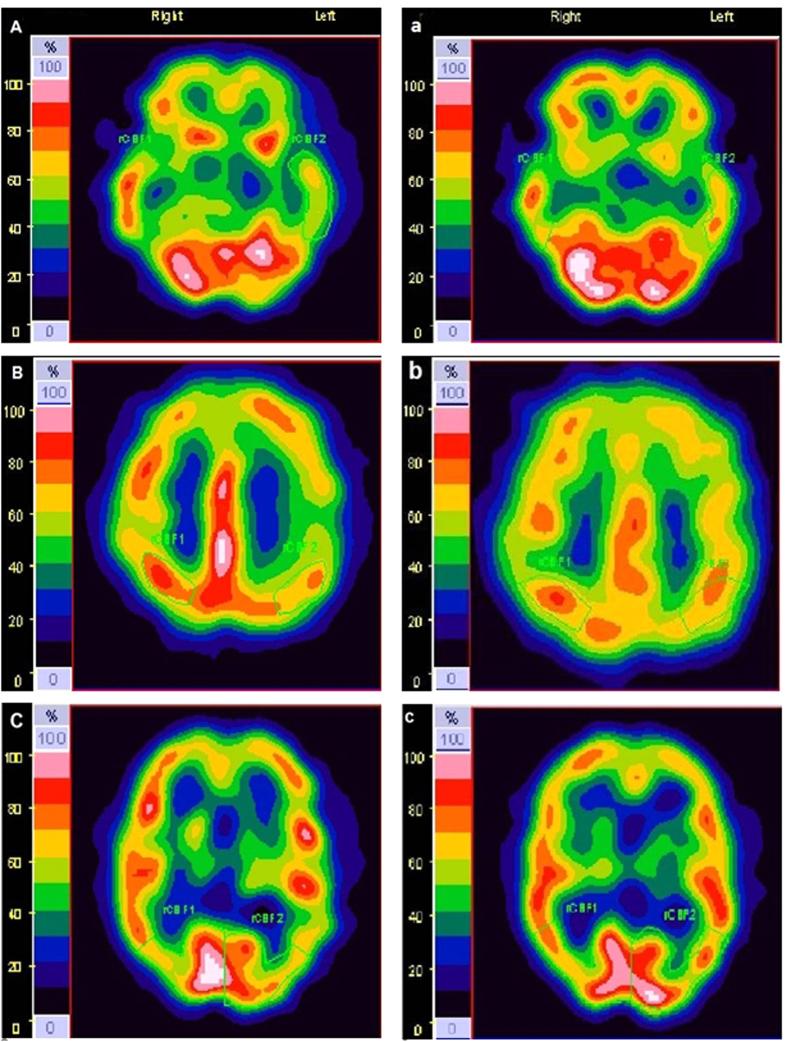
Representative SPECT images before rTMS ((A): temporal lobe; (B) parietal lobe; and (C): occipital lobe), and after rTMS (a–c), showing the areas that displayed a significant increase in rCBF in the patients with ISSHL after the application of low-frequency rTMS treatment over the TAC ipsilateral to the affected ear.
